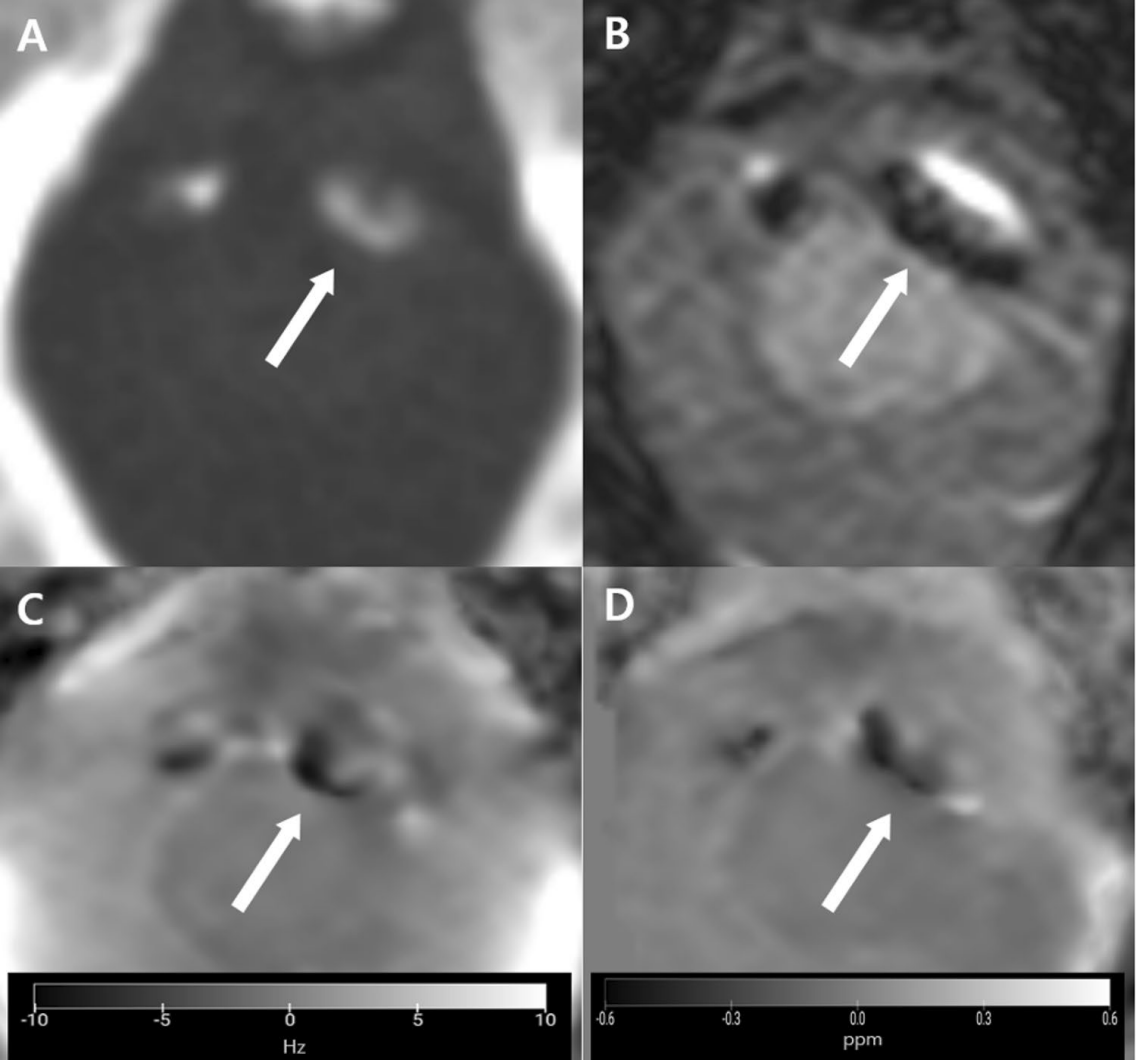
An atherosclerotic calcification in a 68-year-old male patient. (A) An axial precontrast CT at the level of the foramen magnum shows wall calcification in the left intracranial vertebral artery (arrow). (B) A magnitude image shows a dark signal intensity of the lesion (arrow). (C) A phase image shows a heterogeneous low signal intensity lesion of the lesion (arrow). (D) The lesions show low susceptibility values (mean − 0.257 ppm; median − 0.265 ppm) on quantitative susceptibility mapping (QSM) (arrow), suggesting the diamagnetic nature of the calcification. The figures were generated using the MRIcroGL version 1.2.20220720 (https://www.nitrc.org/projects/mricrogl/).
